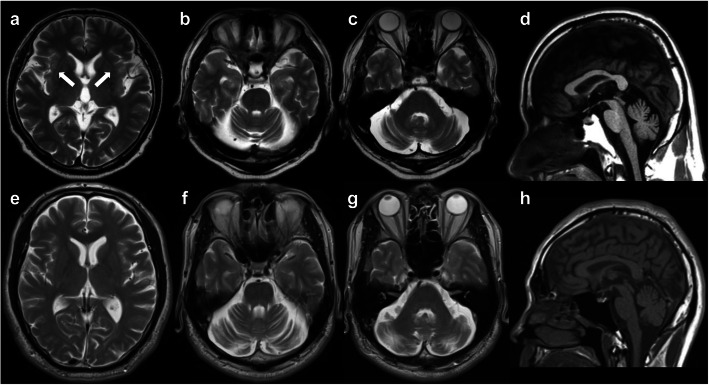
Brain MRI. (a–d): Patient II-1 in Family 1 had mild atrophy of the cerebellar hemisphere and vermis. A hyperintense lateral putamen rim (arrows) was seen. (e–h): Patient II-1 in Family 2 had moderate atrophy of the cerebellar hemisphere and vermis. No brainstem atrophy, hot cross bun sign, or atrophy/hyperintensities in the middle cerebellar peduncles was observed in either patient
